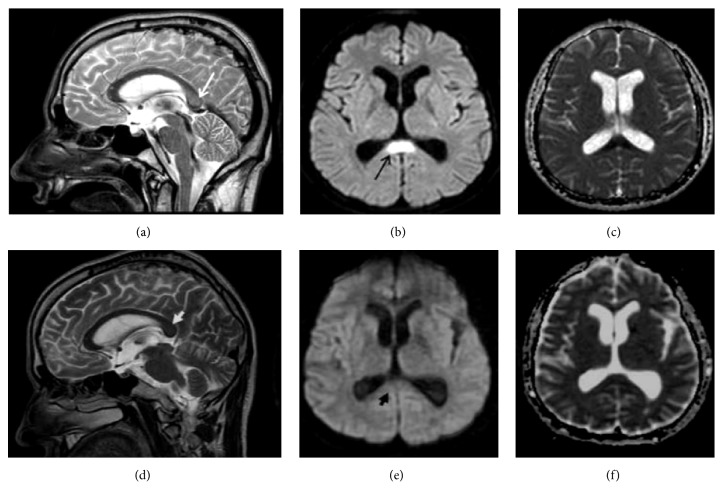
MRI studies at onset (a-c) and 1-month follow-up (d-f). Sagittal FSE-T2 image (a) demonstrating hyperintense regions in the splenium of the corpus callosum (*white arrow*). DWI (b) image shows its hyperintense signal (*black arrow*) and ADC map (c) shows its reduced diffusivity. Corresponding sagittal FSE-T2 image (d) and DWI images (e) showing normal signal in the splenium of the corpus callosum (*white arrow head *and* black arrow head*, respectively) where the ADC map (f) shows normalization of water diffusivity.
